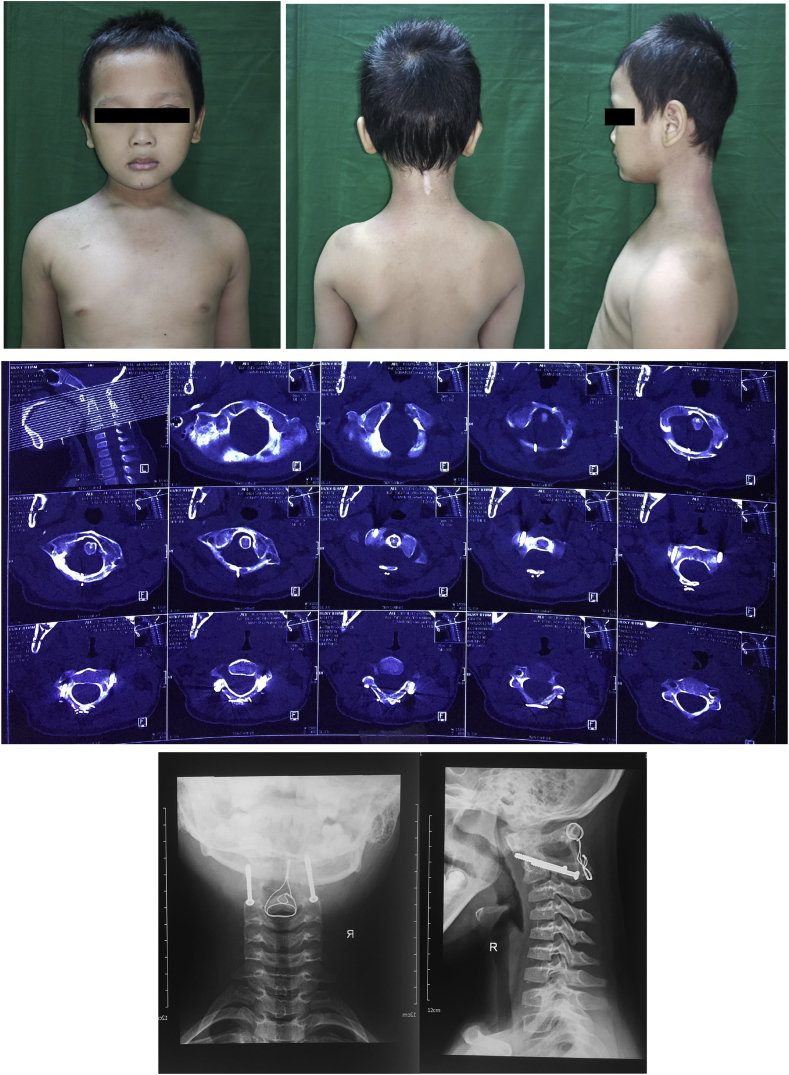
Clinical image, CT findings, and plain radiographs six.
